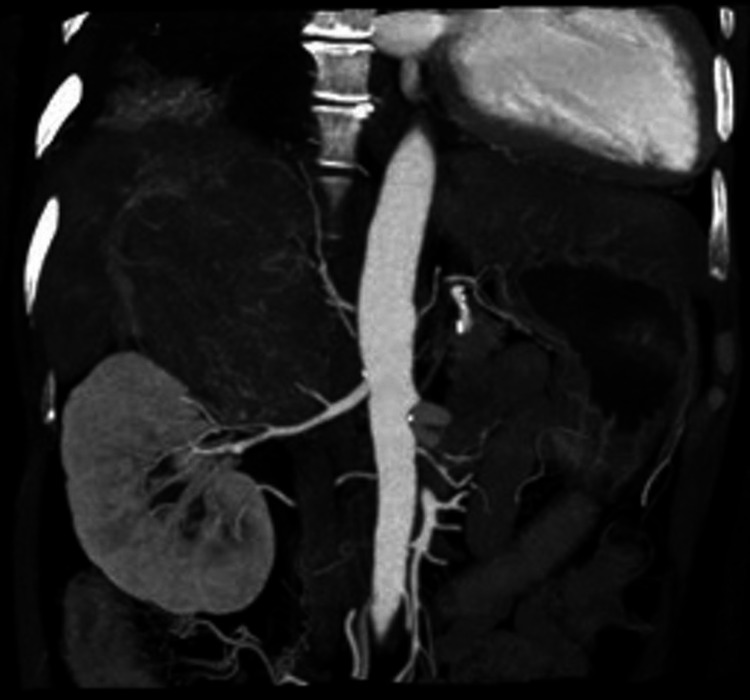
CT whole abdomen coronal view with contrast media
